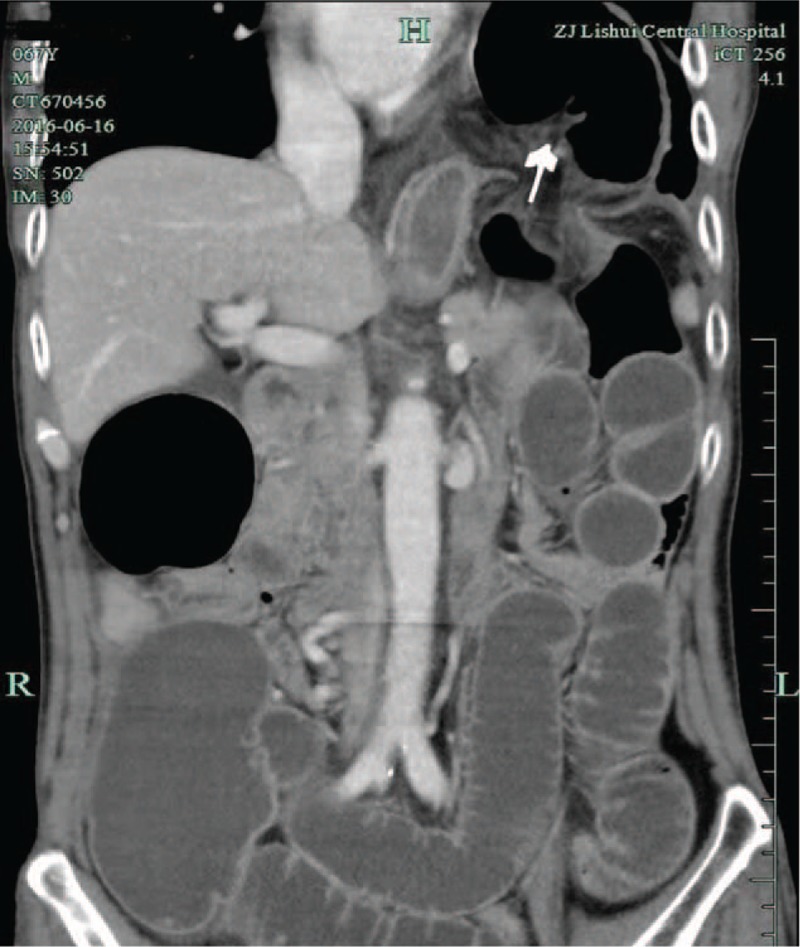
A CT scan of the abdomen was showed marked left diaphragmatic hernia formed (as shown by the arrow). CT = computed tomography.
